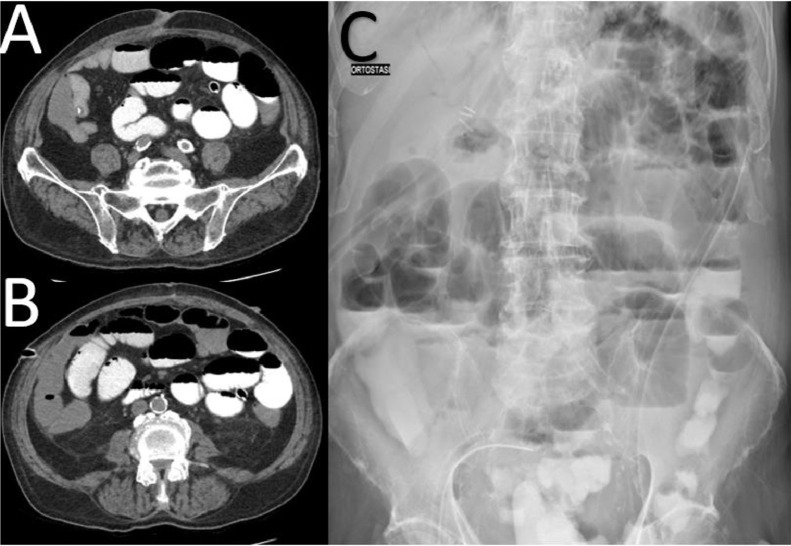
Oral contrast CT showing fluid and fluid-gas distensions in the ileum (A, B), with contrast passing beyond the ileocecal valve (C). Mild fluid distention of the right colon and sigmoid colon was also noted (C).
